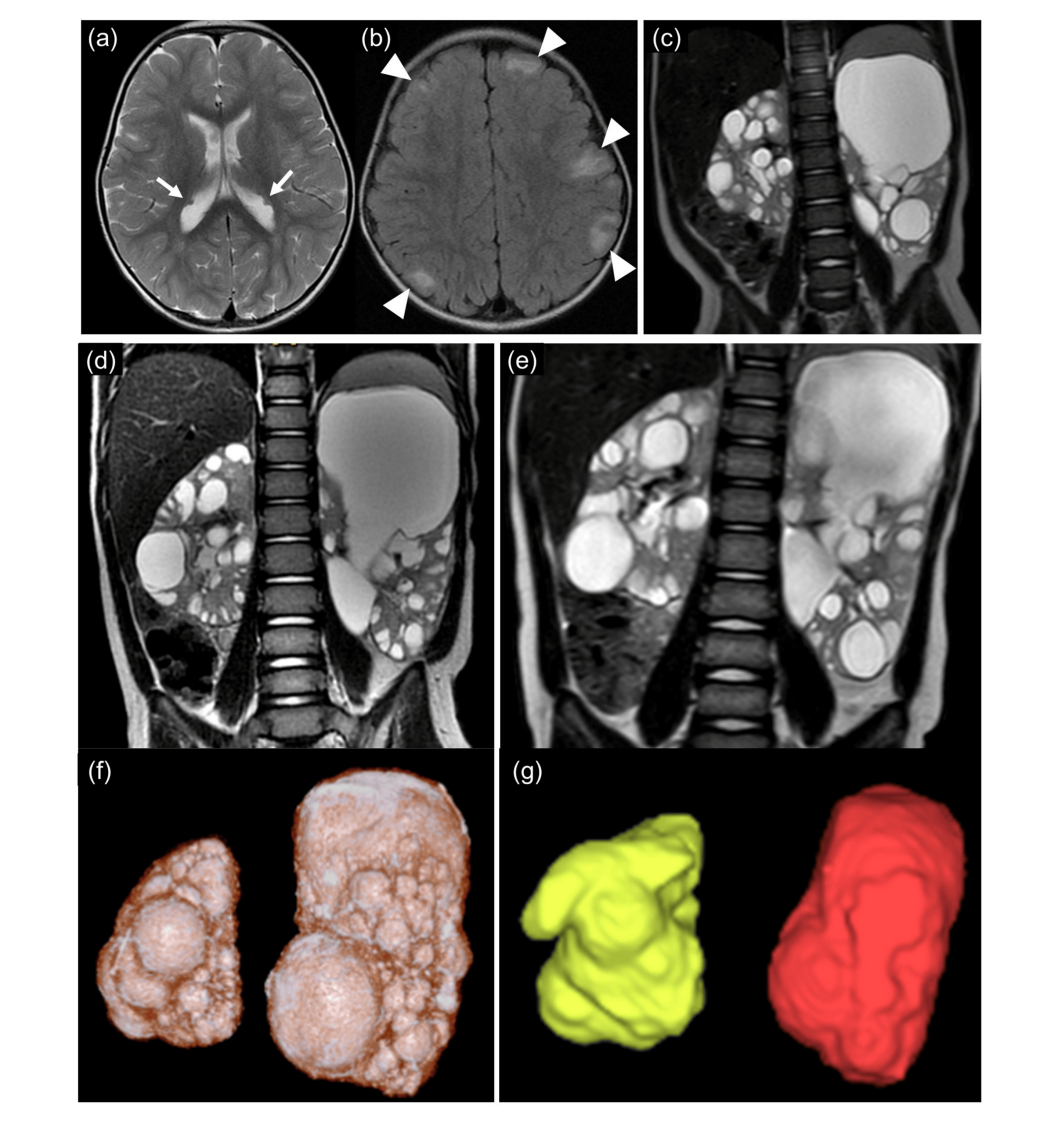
Imaging of an infant with TSC2/PKD1 contiguous gene syndrome. (a) Axial T2-weighted magnetic resonance imaging (MRI) of the head at 1 year and 9 months of age revealing hypointense subependymal nodules in the posterior of the lateral ventricles (arrows). (b) Axial fluid-attenuated inversion recovery image revealing multiple cortical tubers (arrowheads). (c) Coronal T2-weighted abdominal MRI at 1 year and 10 months of age revealing asymmetrically enlarged kidneys with polycystic lesions of various sizes. (d) Coronal T2-weighted abdominal MRI at 2 years 7 months of age and (e) at 3 years 9 months of age. (f) The kidney size volumetry; right: 194 ml, left: 546 ml at 2 years 7 months of age and (g); right: 287 ml, left: 559 ml at 3 years 9 months of age. These revealed gradual enlargement of the kidneys and advancement of polycystic kidney disease.
